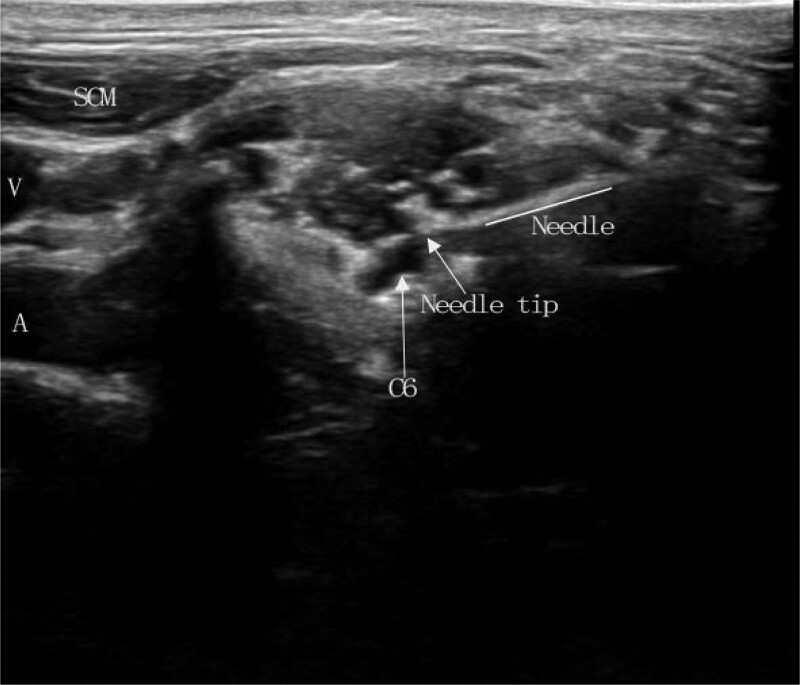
Ultrasound-guided pulsed radiofrequency of the left cervical 6 nerve root. C6 = the left cervical 6 nerve root, SCM = sternocleidomastoid muscle, V = jugular venous, A = carotid artery.
